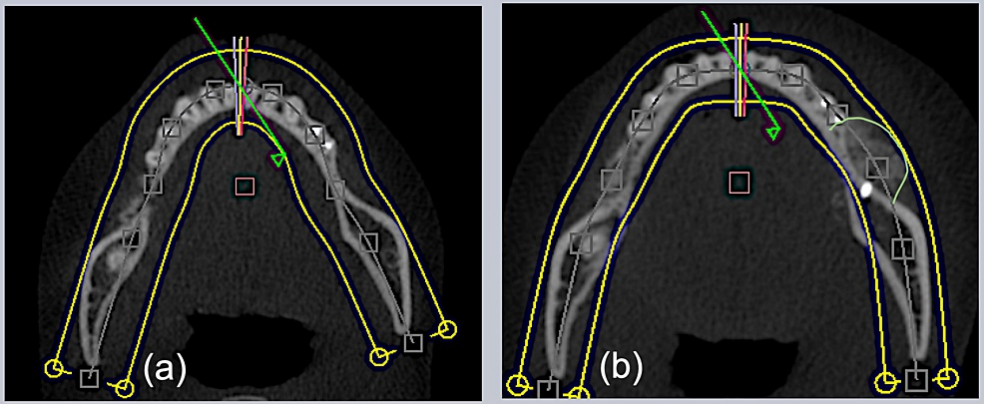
Cone beam CT occlusal view comparison. (a) Before; (B) after.
